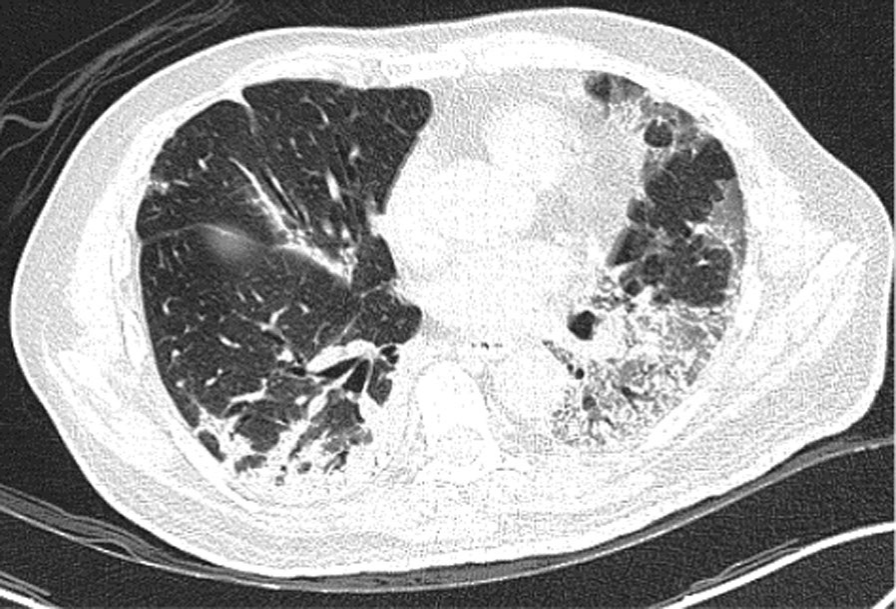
CT shows areas of fibrosis, bands, and dilatation of lower lobe bronchioles on left side indicative of stage of resolution
